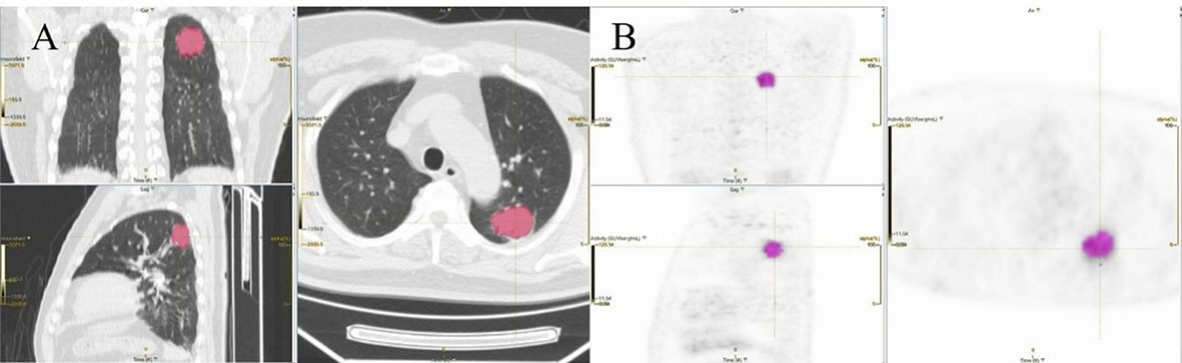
An example of segmentation coronary image, sagittal image, and transaxial images of CT (A) and PET (B) showing an example of VOI for measuring imaging features of NSCLC.
